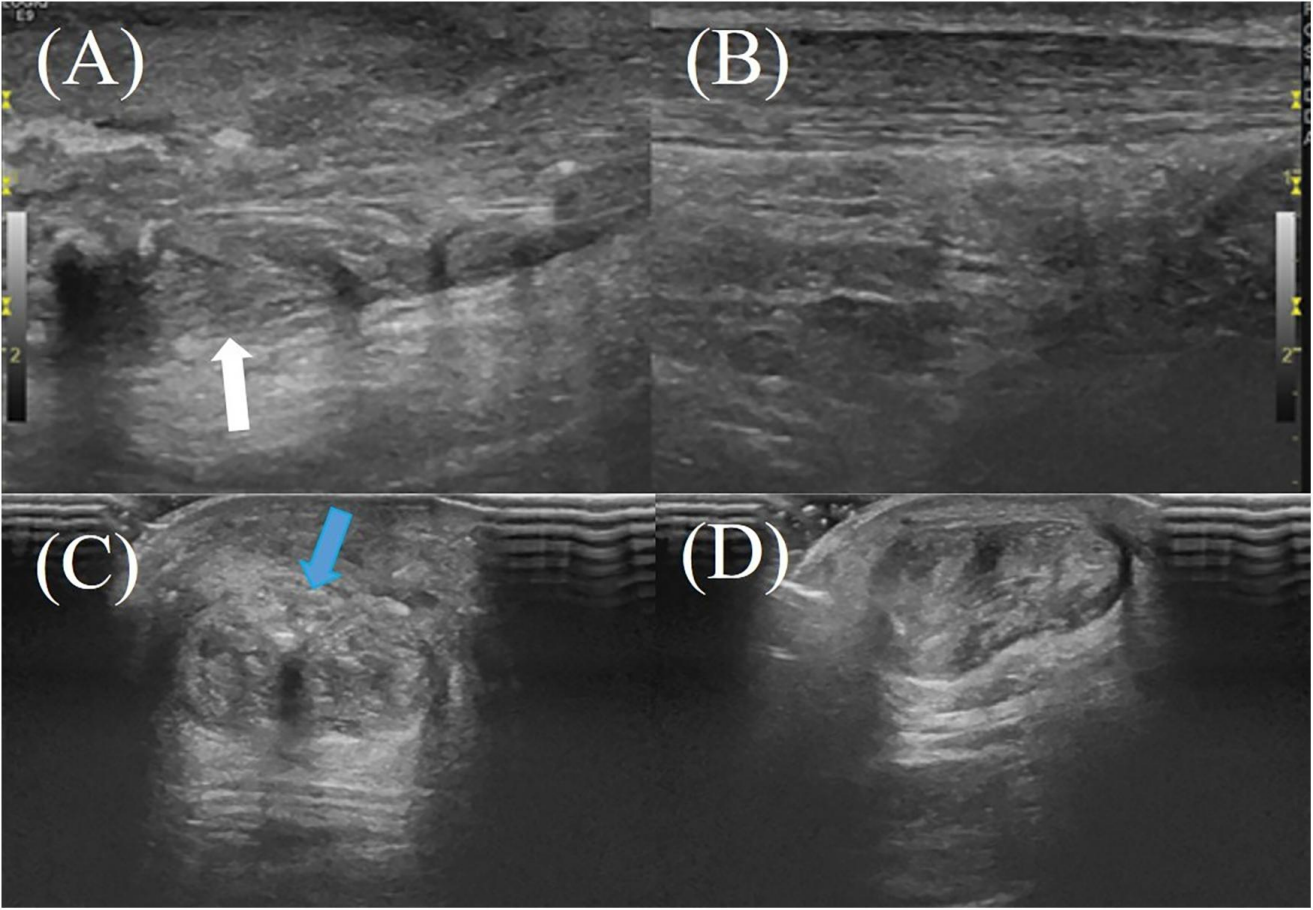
A 61-year-old male patient with right Achilles tendon rupture located 3.0 cm proximal to the calcaneal insertion underwent surgical repair without amniotic membrane augmentation. At the 2-month postoperative follow-up, ultrasound evaluation revealed: (A) the repaired tendon on the right side (white arrow) demonstrating heterogeneous echotexture on the longitudinal plane; (B) the healthy contralateral (left) Achilles tendon on the longitudinal plane; (C,D) the repaired right Achilles tendon on the transverse plane, showing irregular hyperechoic signals near the superficial tendon surface, indicating tendon fibrosis or degenerative changes (blue arrow).
